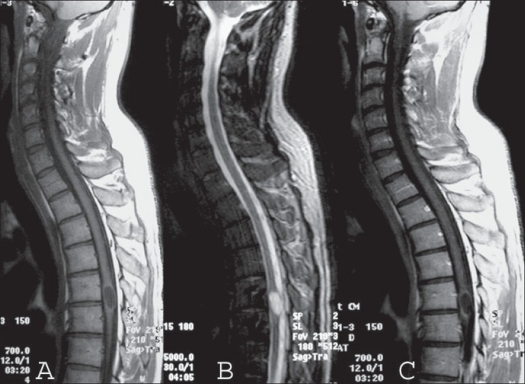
Mid sagittal T1 WI (A) of MRI of cervico dorsal spine shows a hypointense intramedullary lesion at D4 level, which became hyperintense on T2 WI (B) sequence with mild enhancement at periphery with gadolinium contrast (C)
